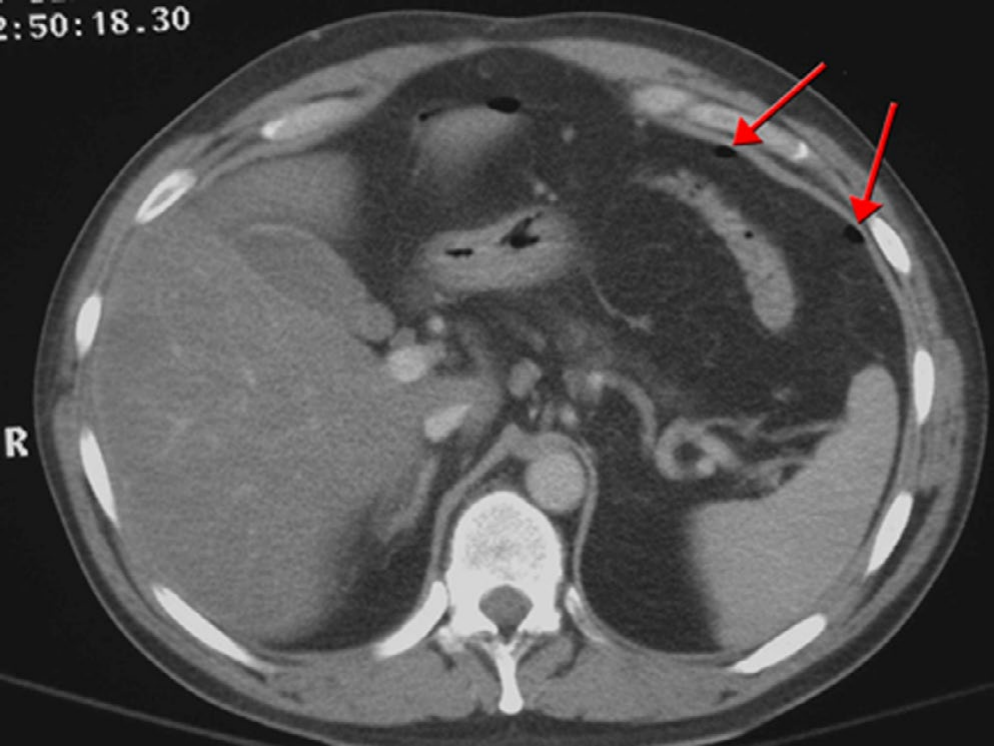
Image from CT scan of abdomen
